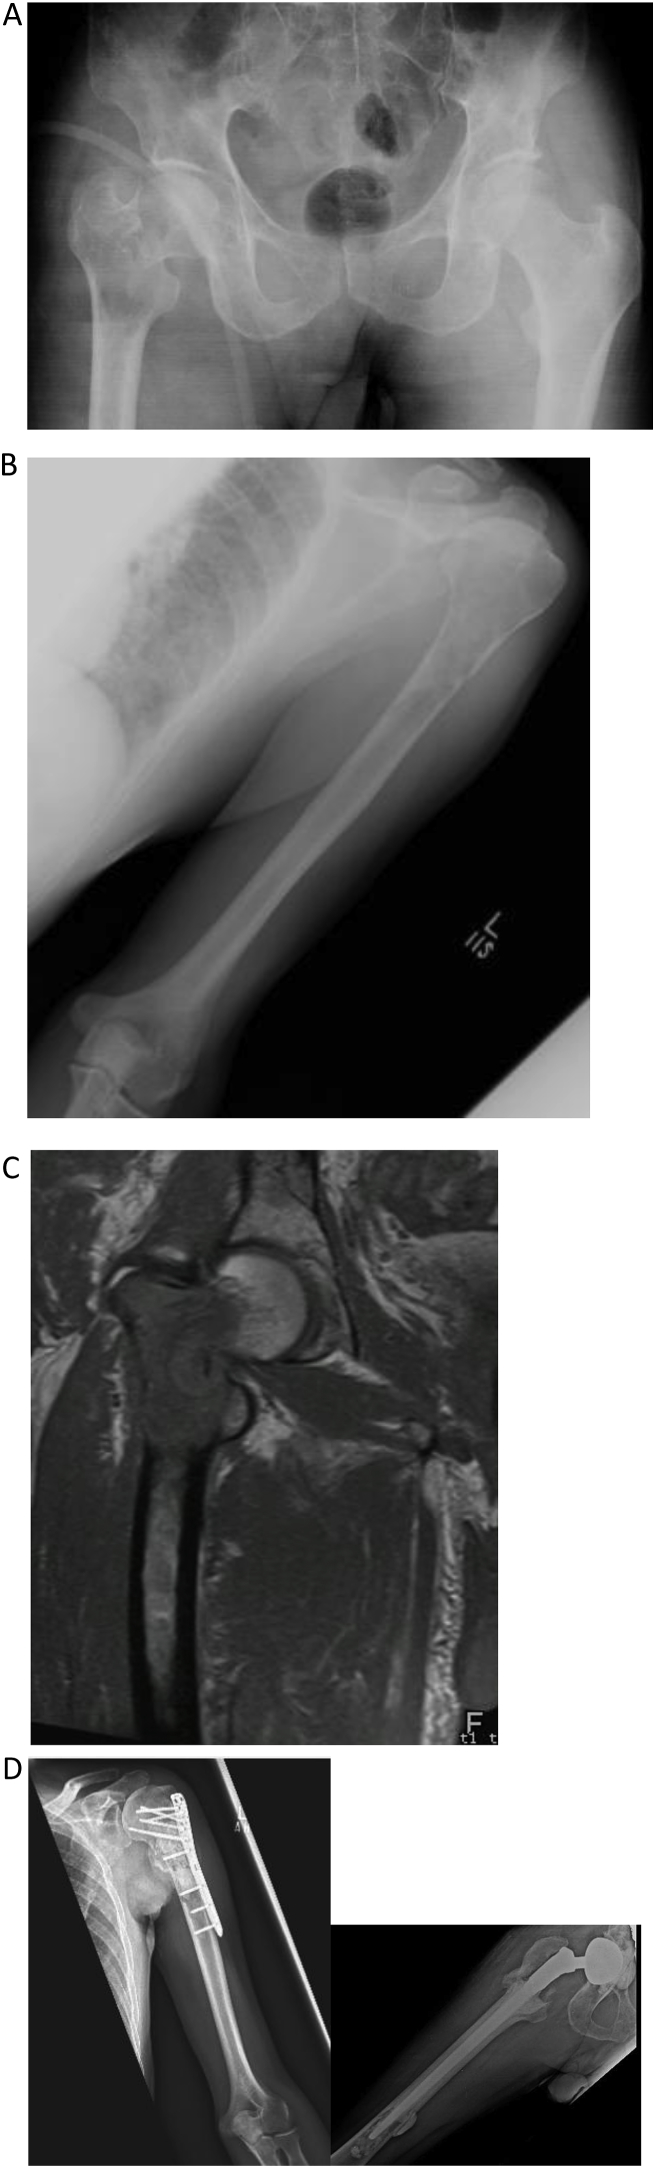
45-year-old male, known to have multiple myeloma, presented with pain in left shoulder and right hip. A: x-ray Pelvis showing pathological fracture right Femur. B: X-ray left Humerus with lytic lesion. C: MRI showing fracture and the extent of the lesion in the proximal Femur. D: ORIF left proximal Humerus with cement and right-sided proximal femur replacement with THR with dual mobility acetabular cup was performed for complete pain relief and function.
